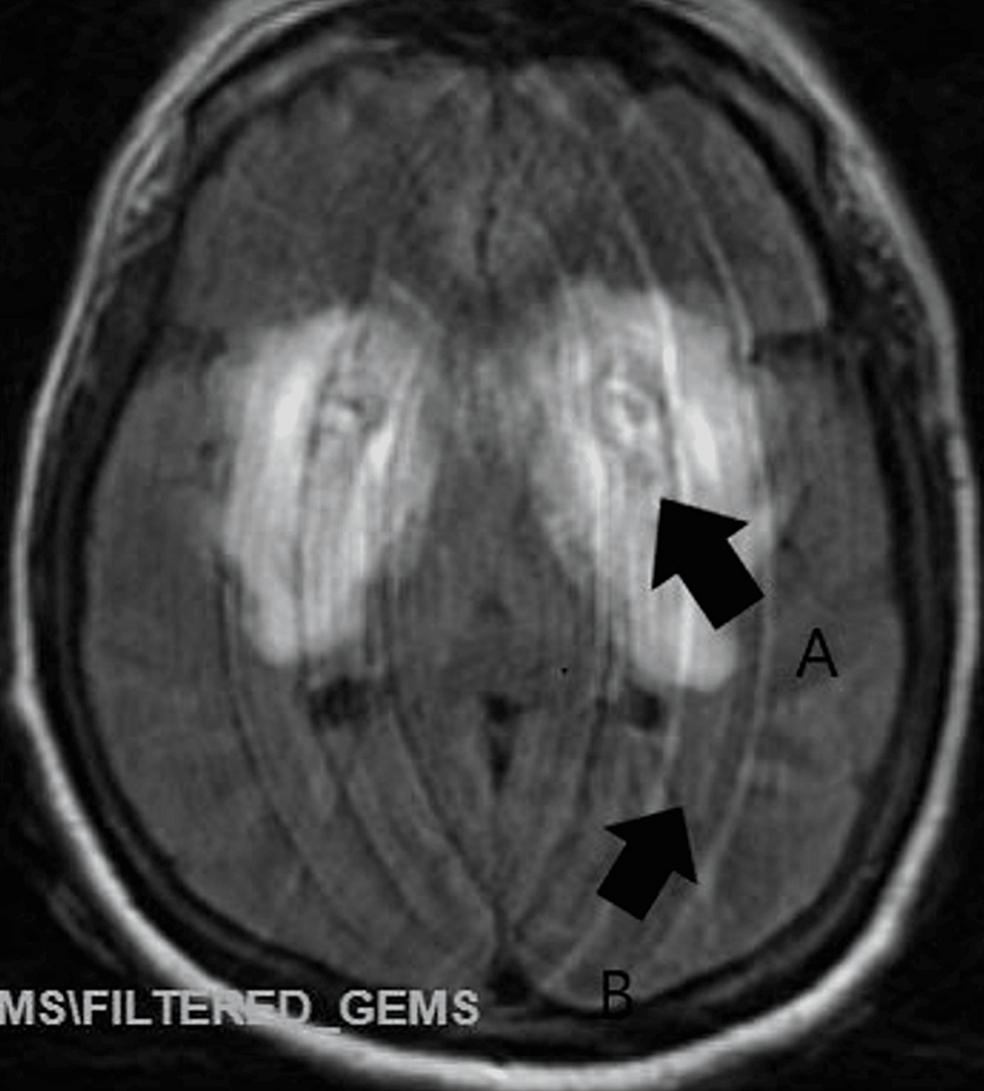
Initial MRI without contrast revealing concomitant infarction and hemorrhaging of the bilateral lentiform nuclei. A limited multiplanar/multisequence MRI of the brain was performed without contrast. The figure was obtained from FLAIR AX imaging. Limited exam due to motion degradation, demonstrating restricted diffusion (Arrow A) within bilateral lentiform nuclei suggestive of infarction as well as hemorrhage with associated marked edema (Arrow B) in the adjacent brain parenchyma suspicious for hypoxic-ischemic changes. No hydrocephalus was seen.
